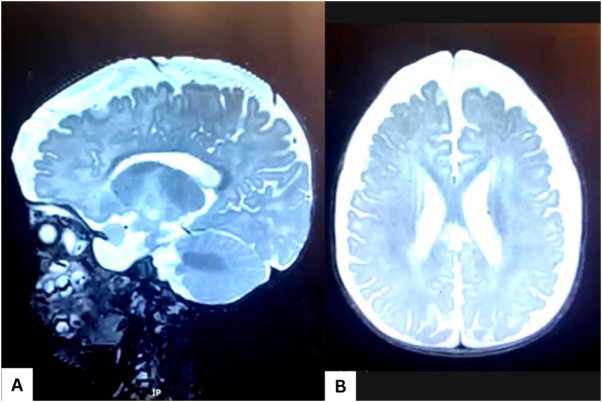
Brain MRI scans at the diagnosis of optic glioma. (A) Sagittal T2 scan; (B) Axial T2 scan.
